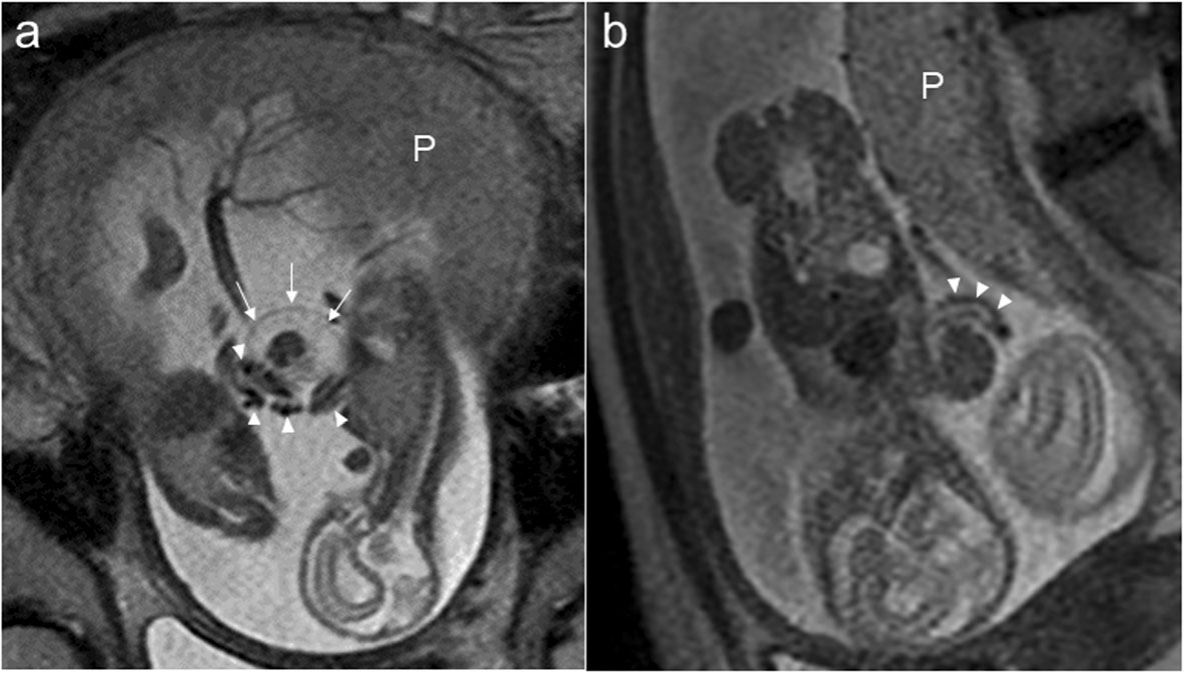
Magnetic resonance imaging. Magnetic resonance imaging at 18 weeks of gestation. The upper left arm of baby B is swollen, and edema is present (a, arrows). The umbilical cord is seen encircling the same arm (a, b, arrowheads)
